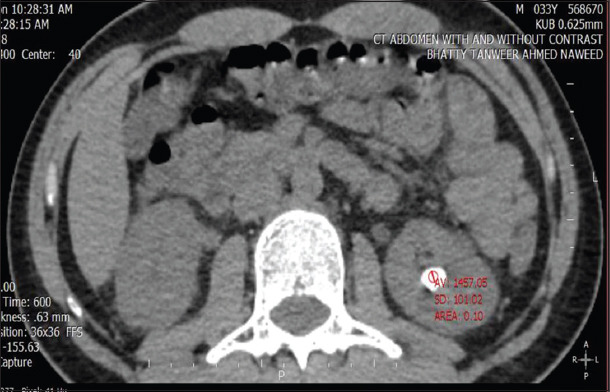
Mean stone density and standard deviation of stone density (also called stone heterogeneity index) in red elliptical area on axial view of computed tomography scan.
